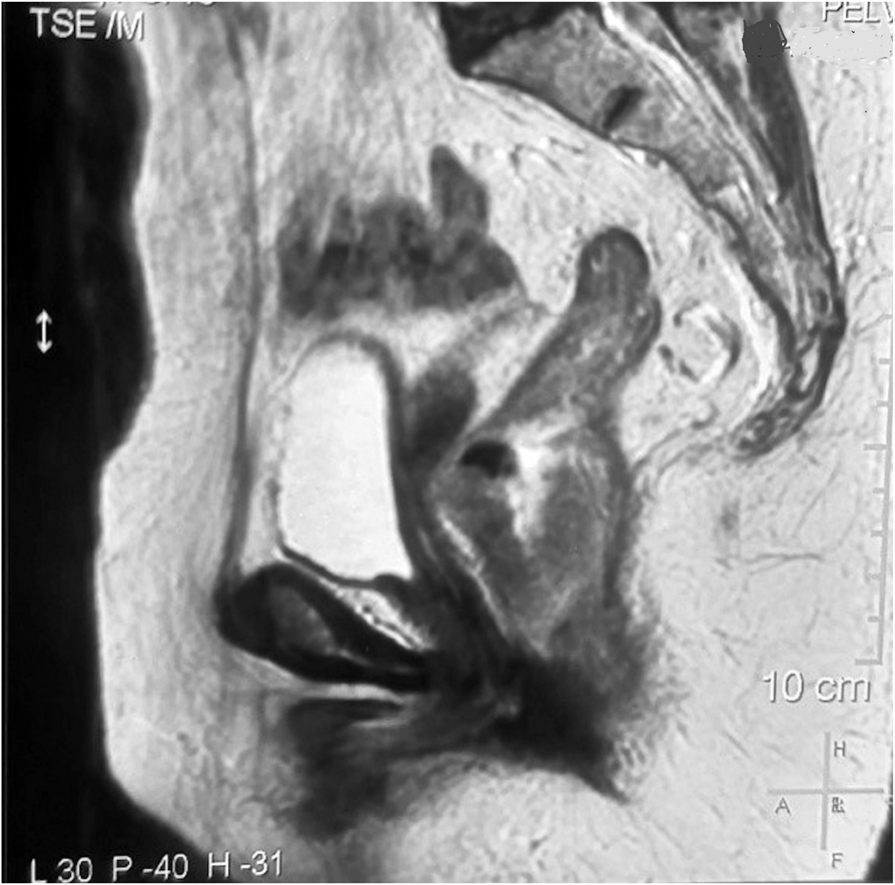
Sagittal view of MRI pelvis. Lesion extends from 26 mm proximal to anal verge and measures 52 mm in cranio-caudal dimension, planes with urinary bladder and uterus well maintained
